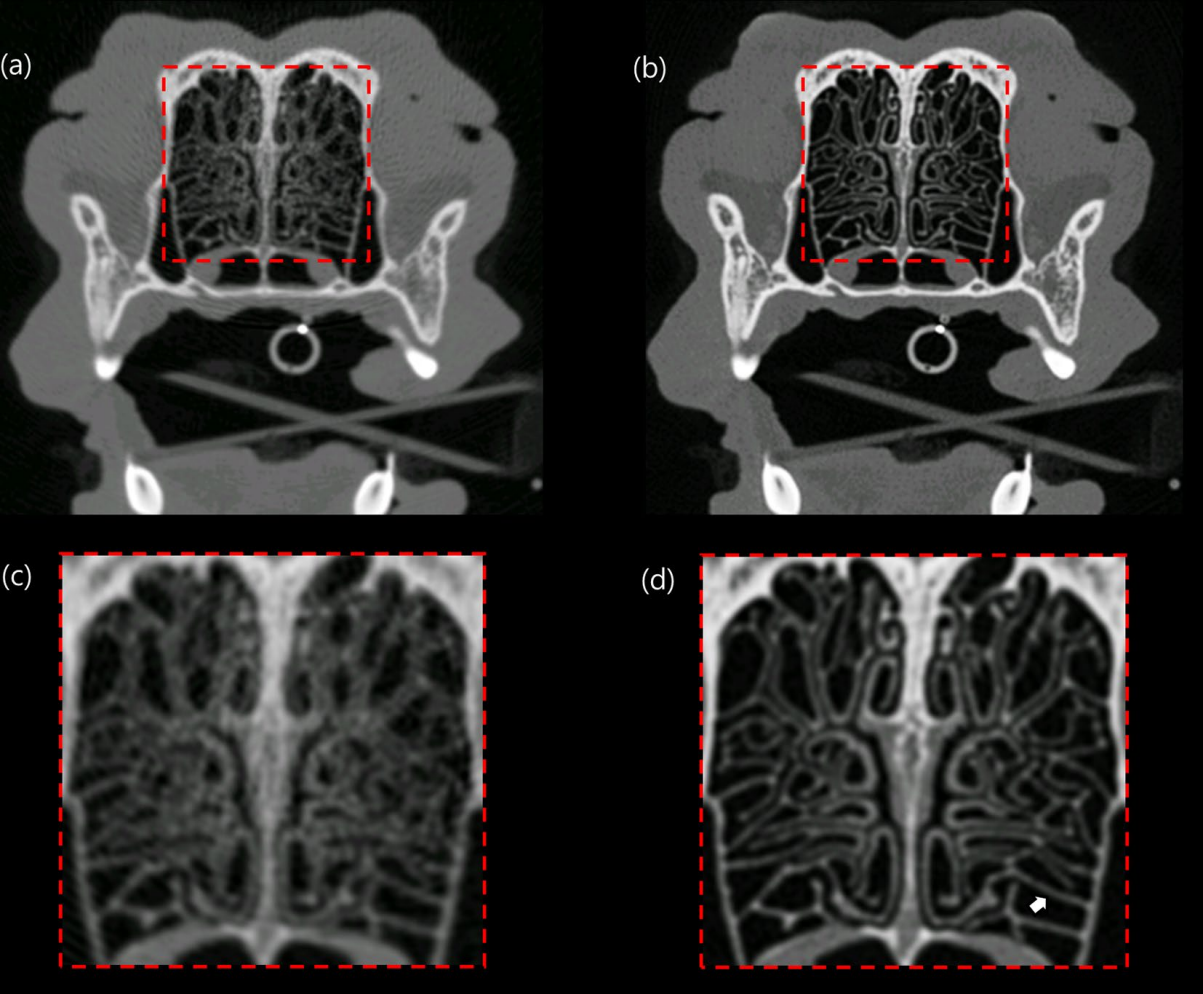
Axial images of the nasal turbinates scanned with the use of the (a) standard mode and (b) high-resolution (HR) mode of the PCD CT system. The average thickness of nasal turbinates (white arrow) is 0.5 mm. (c, d) The zoomed-in ROIs show better delineation of sub-millimeter nasal turbinates using (c) the HR modecompared with (d) the standard mode.
